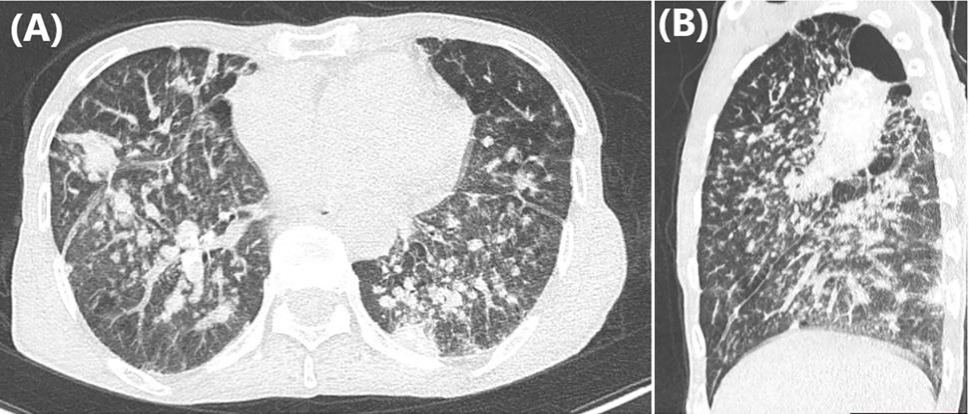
chest computed tomography scan in parenchymal window on axial (A) and sagittal (B) views showing multiple bilateral peri-lymphatic parenchymal and subpleural nodules with pseudo-plaques predominant in the upper lobes
